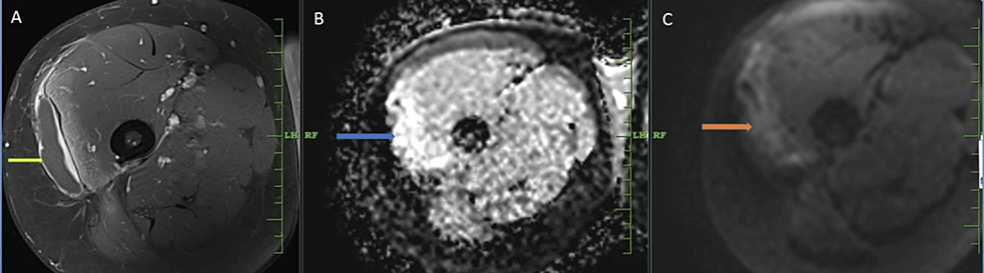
MRI of the right thigh showing collection. (A) Post-contrast T1-weighted axial image showing peripherally enhancing collection(yellow arrow) adjacent to the iliotibial band. (B) ADC image and (C) diffusion image, no diffusion restriction was noted within the collection (blue arrow in B, orange arrow in C). MRI, magnetic resonance imaging; ADC, apparent diffusion coefficient
